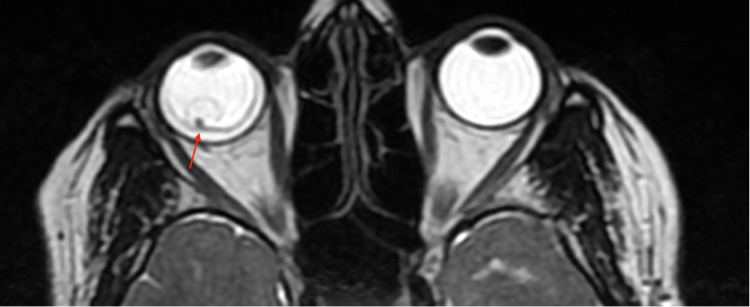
MRI of the T2W axial section showing cyst in the right eye
